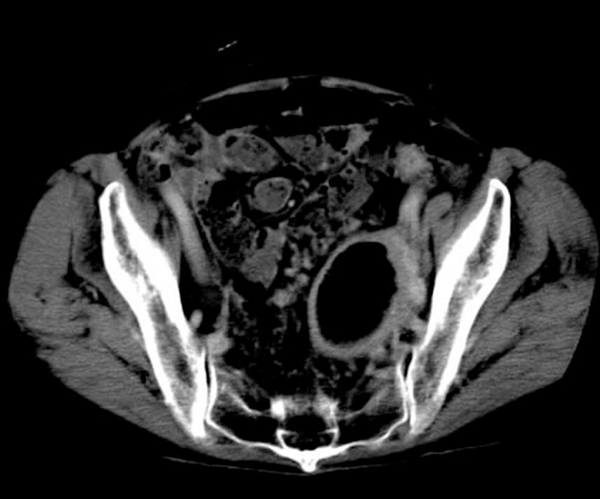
Computed tomography revealed the capsular lesion including air density with a diameter of 5 cm between the posterior wall of the uterine body and the sigmoid colon.
